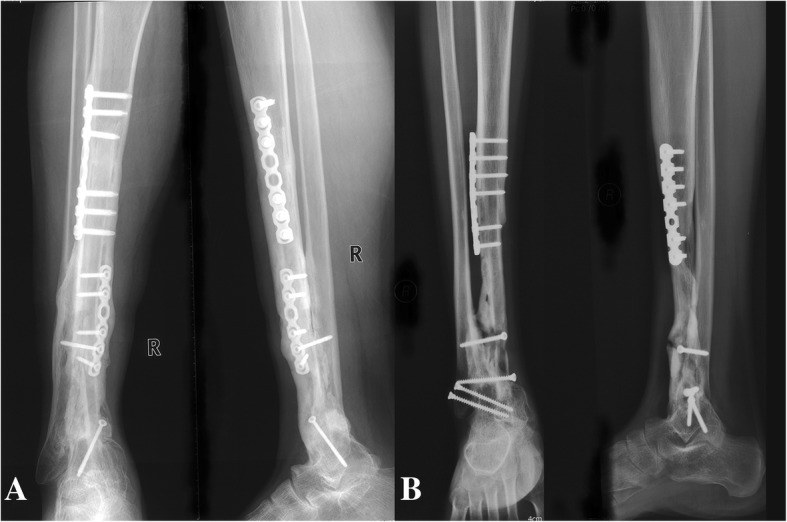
a The radiograph obtained at 30 months after surgery showing the fusion of the graft and host bone (Patient 1). b Postoperative 24 months radiograph showing successful fusion in both proximal and distal junctions (Patient 5)
